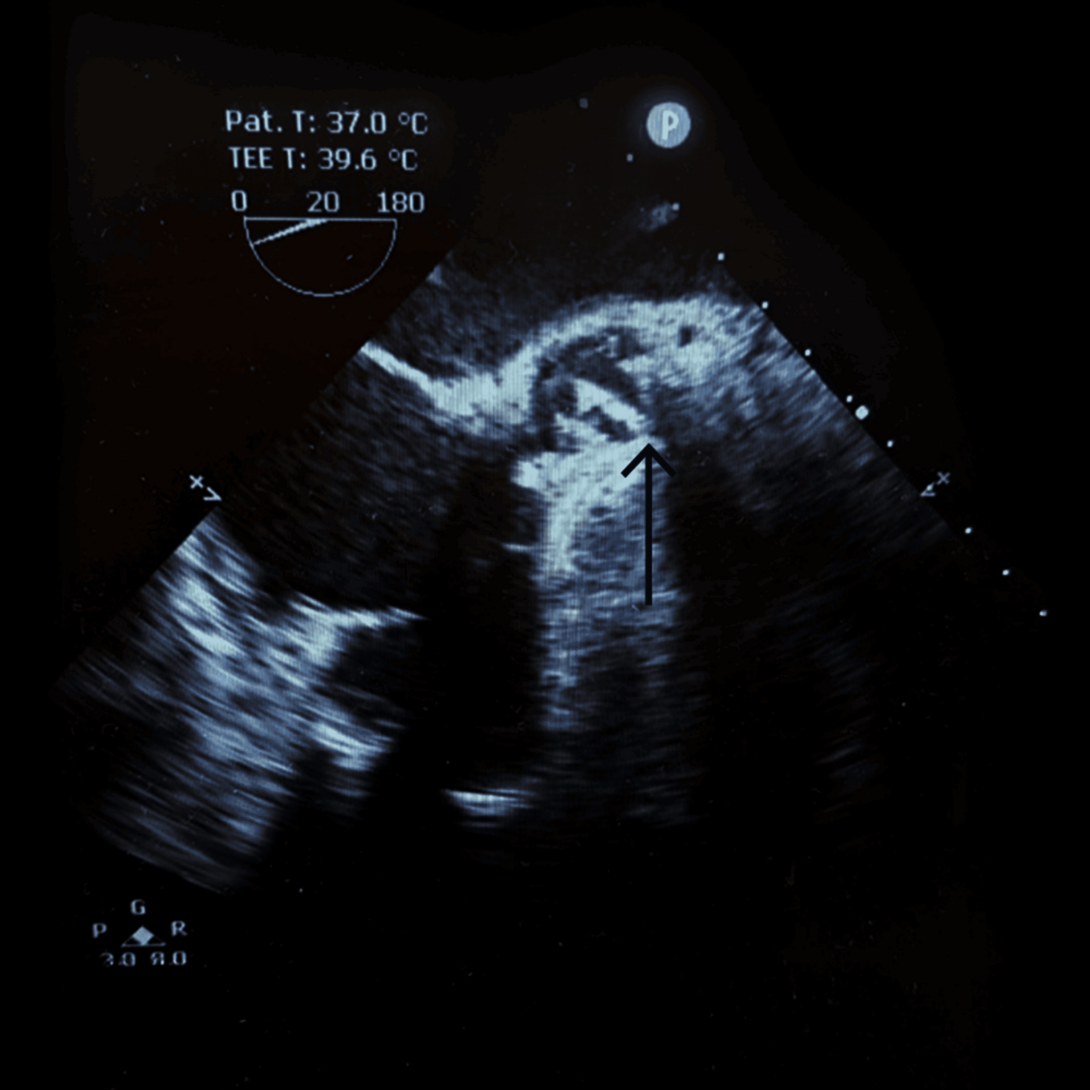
Intraoperative transesophageal image of the aortic valve in situ.
